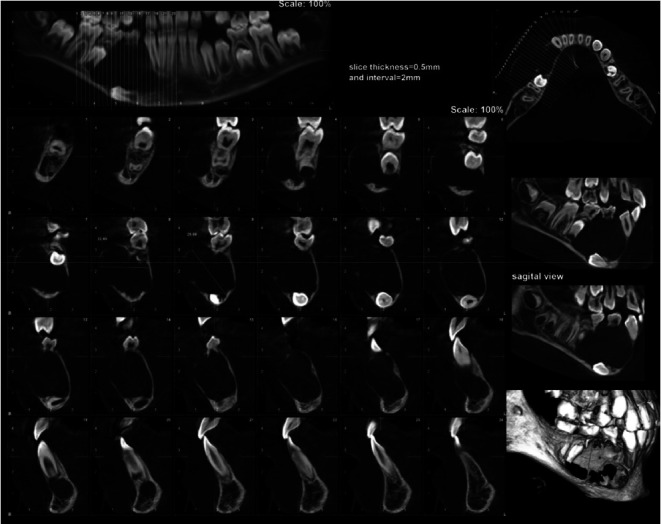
Cross-sectional view of CBCT (cone beam computed tomography) shows expansion of the lesion, which causes displacement and impaction of permanent first premolar into inferior border of the mandible
